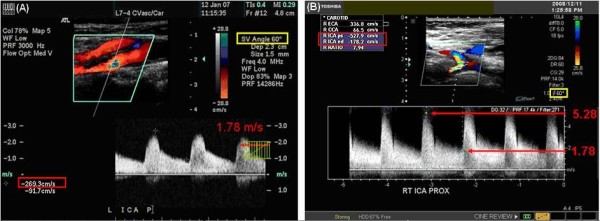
Stenotic ICA Images and Waveforms. A. Systolic velocity > 1.25 m/s is consistent with an angiographic stenosis > 50% diameter reduction (DR). Both the PSV = 2.69 m/s and the EAV = 1.78 m/s exceed the criterion. Color aliasing (cyan/blue) can be seen at the stenosis location, although the color aliasing velocity of 0.29 m/s, when adjusted for Doppler angle of 60 degrees (0.58 m/s) and for estimated aliasing magnitude (1.16 m/s), suggests that the color image was captured in diastole, at the right edge of the waveform. B. EDV > 1.4 m/s is consistent with a severe angiographic stenosis > 80% DR. A magnified view of the color flow lumen image appears to reveal no hemodynamic stenosis. The apparent filling of an extra-luminal space with color is sometimes due to specular (mirror-like) reflection of ultrasound from the deep luminal surface. If the extravascular tissue is echogenic, write priority will suppress the Doppler color, but if the extravascular tissue is anechoic, Doppler color remains in that portion of the image. The dark (anechoic) region between the color flow lumen and the brighter arterial wall deep to the Doppler color would be interpreted by some as plaque burden.
